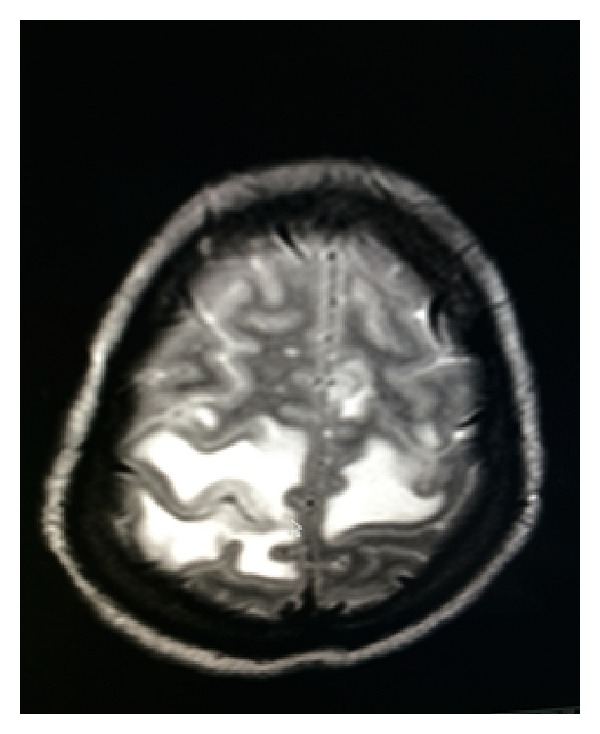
MRI-T2-Axial View: bilateral parietal vasogenic edema.
